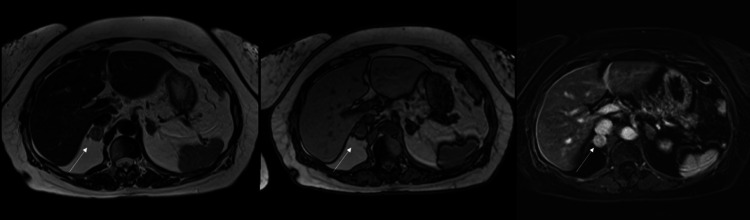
MRI abdomen showing lesions in the right adrenal gland in T2, out-of-phase T1, and T1 C+ (Gd) MRI: magnetic resonance imaging; Gd: gadolinium
